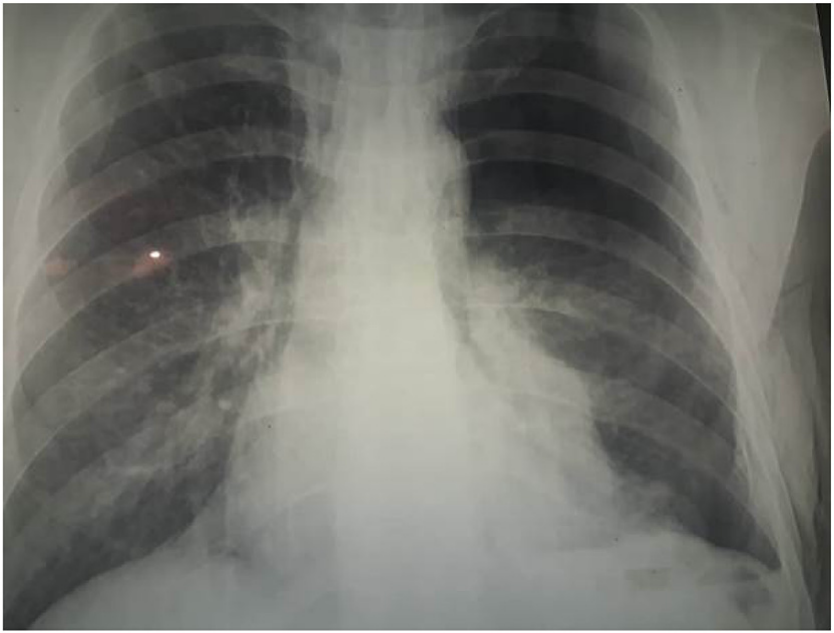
Chest X-ray tomography detection of hemopneumothorax on the right side.
